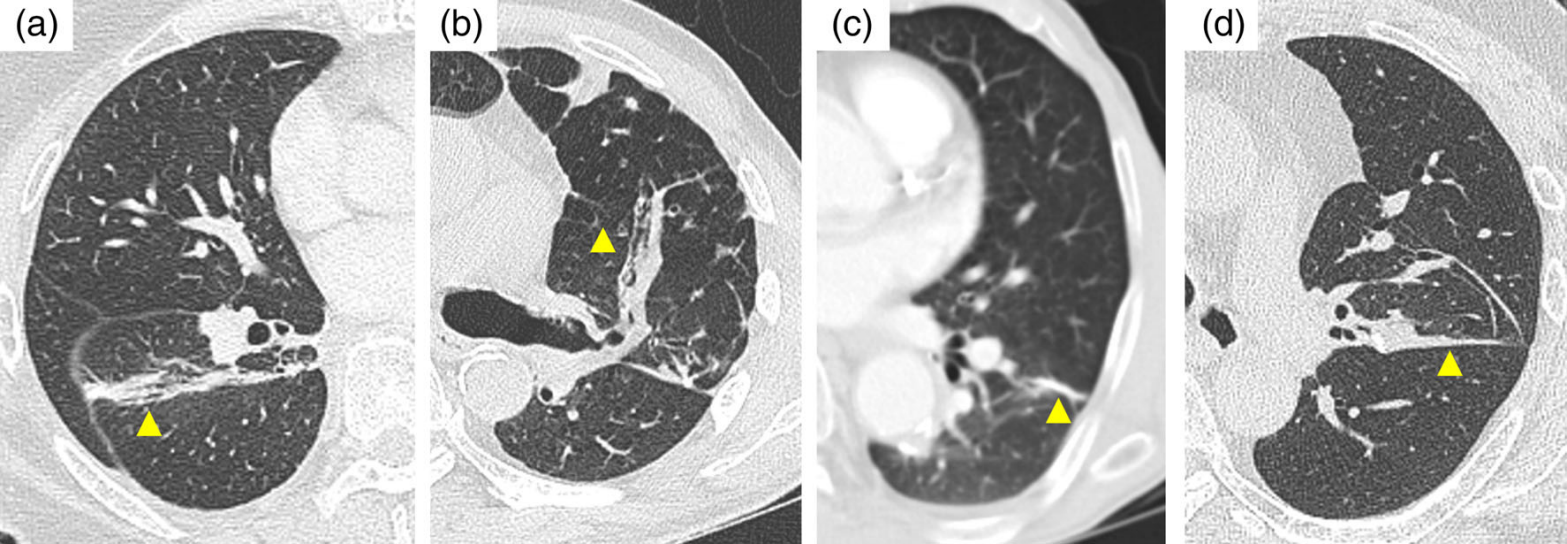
Computed tomography imaging of lung plication after segmentectomy. After nonanatomical segmentectomy, many cases had thicker and wider lung plication, as shown in (a) and (b). (c) and (d) were common findings mostly after anatomical segmentectomy.
